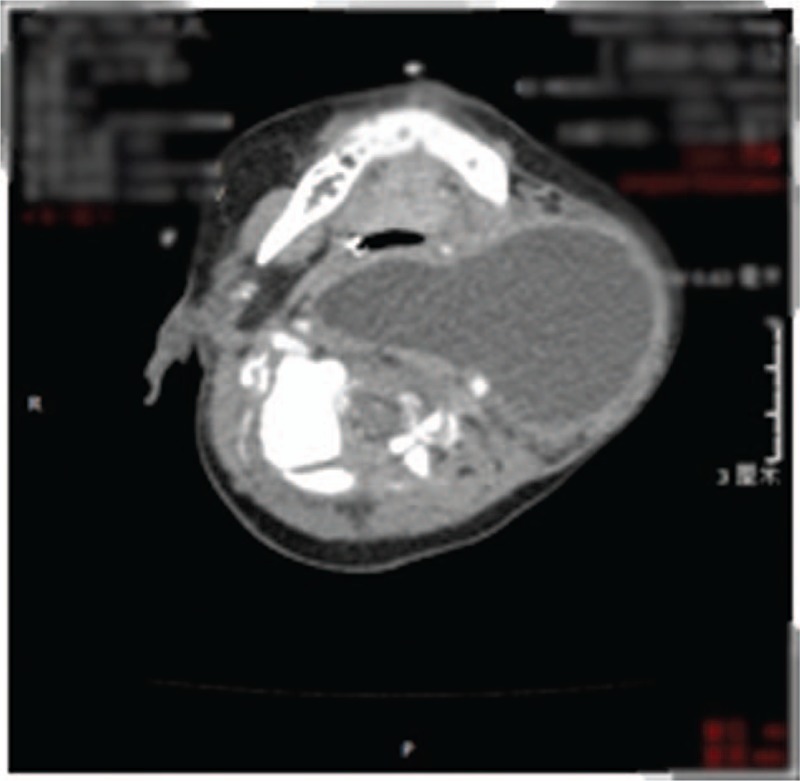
Axial contrast-enhanced CT scan image showing an abscess cavity with an irregularly enhanced cystic wall.
